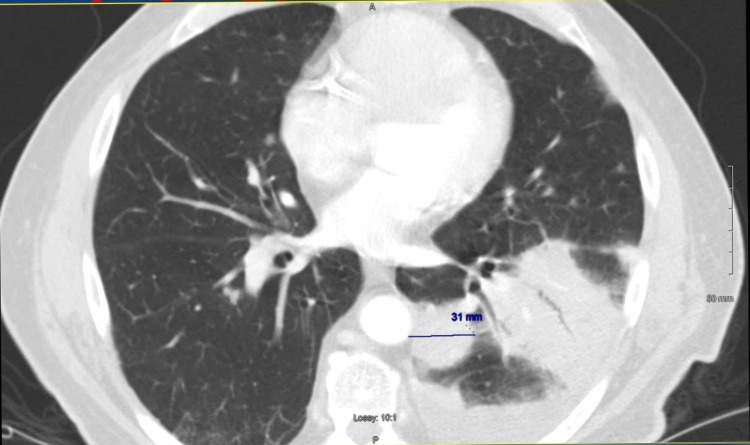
Computed tomography (CT) of the chest showing a lung mass measuring 3.1 cm
